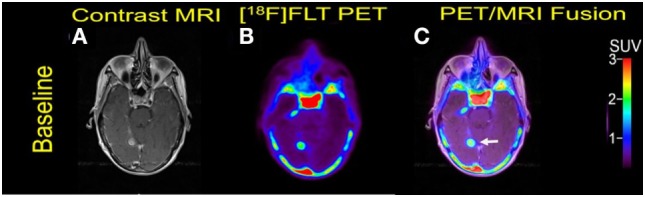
Case #3. Baseline magnetic resonance imaging (MRI) (A), fluorothymidine (FLT)–positron-emission tomography (PET) (B), and fused PET/MRI (C). The 1.1 cm right posterior, medial temporal lobe lesion (arrow) showed FLT avidity with SUV ratio of 7.5.
